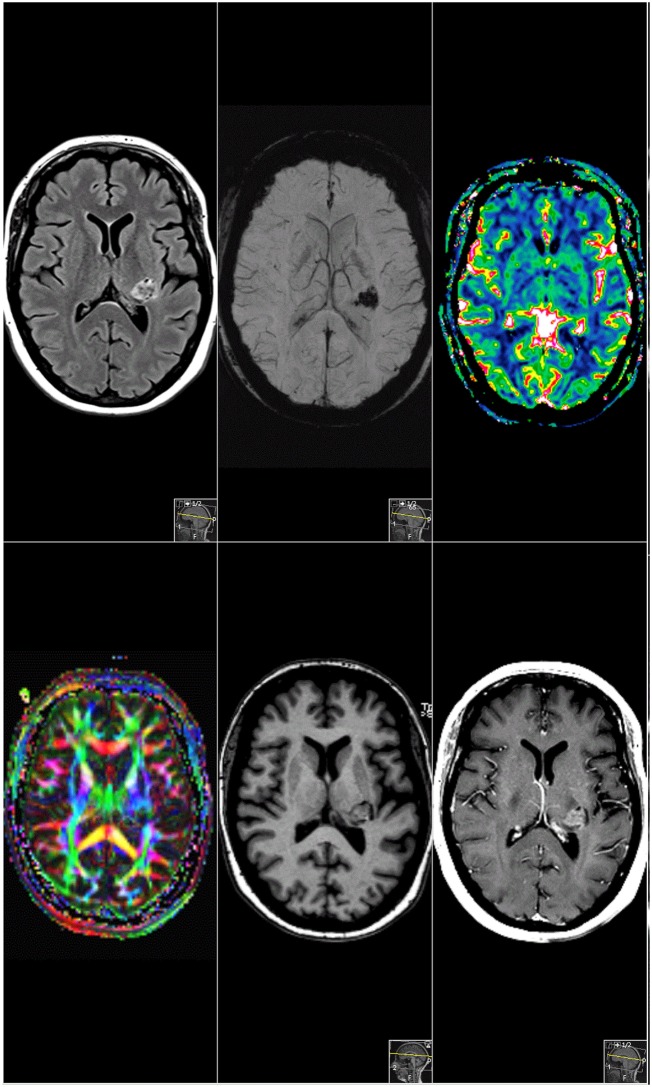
Magnetic resonance imaging. Sagittal cMRI reveals a supratentorial lesion involving the posterior limb of internal capsule, dorsolateral thalamus, partially, the posterior putamen and the corona radiata. The lesion enhanced inhomogenously after gadolinium administration in T1-weighted sequences.
